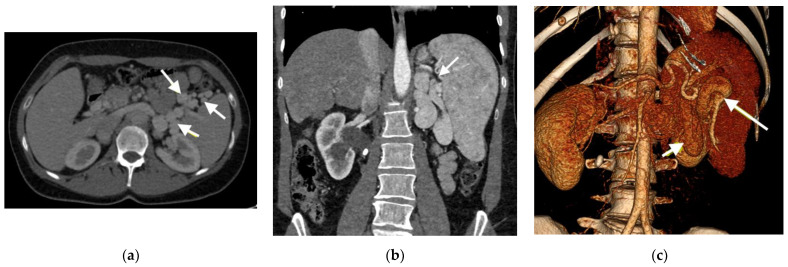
Computed tomography (Patient 2). Arrows show varicose dilatation of the splenic vein: (a) sagittal view; (b) coronal view; (c) 3D reconstruction.
